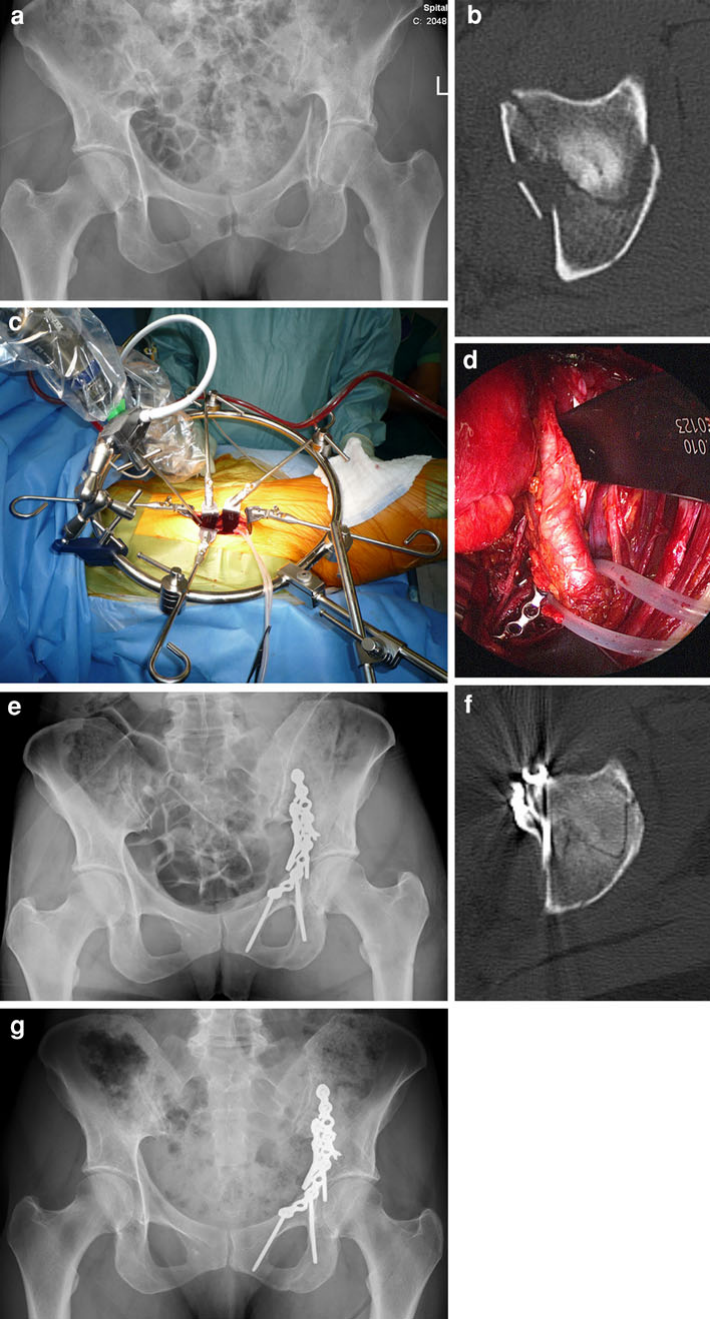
a Anteroposterior radiograph of the pelvis of a 56-year-old female patient after a bicycle accident demonstrates a both-column acetabular fracture with a medialized quadrilateral plate. b Axial CT scan of the pelvis demonstrates a both-column acetabular fracture with a medialized quadrilateral plate. c Intraoperative view of the Pararectus approach and operative setting using the retractor system (Synframe) and endoscope to allow a less invasive approach. d Endoscopic view of the obturator nerve (*left*), reconstruction plates on the pelvic brim and quadrilateral plate, and the mobilized external iliac vessels. e Postoperative anteroposterior radiograph of the pelvis demonstrates anatomic reconstruction of the both column fracture. f Axial CT scan demonstrates anatomic reconstruction of the both-column fracture. g Anteroposterior radiograph of the pelvis 2 years after surgery without any signs of osteoarthritis. The patient showed an excellent clinical result after only 6 months postoperatively and is now working as a yoga teacher
